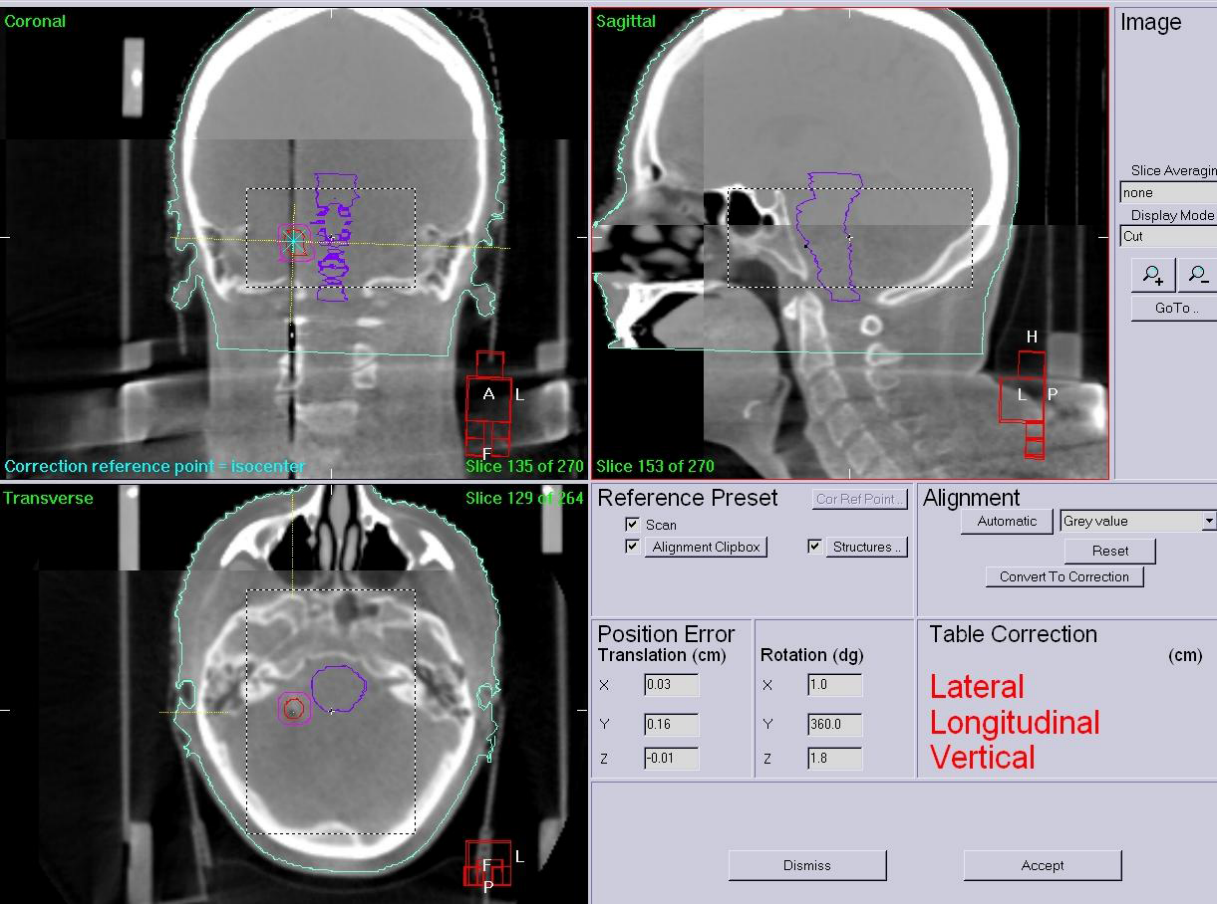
Cone-beam computed-tomography (CBCT) matched with the planning CT using the clip-box involving skull, pneumatic sinusal structure and clivus.
